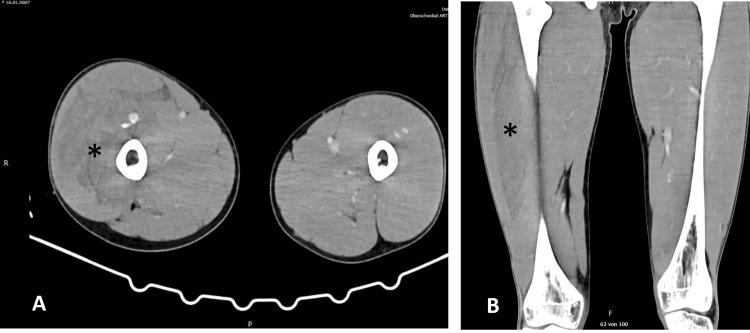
Massive swelling of the right thigh A shows the transverse angiographic CT scan of both thighs, with a clearly increased circumference of the right thigh, visible on the left side of the image. B shows a coronal angiographic CT scan of both thighs, demonstrating edema of the right thigh, appearing as hypodense striae within the quadriceps muscle (*).
